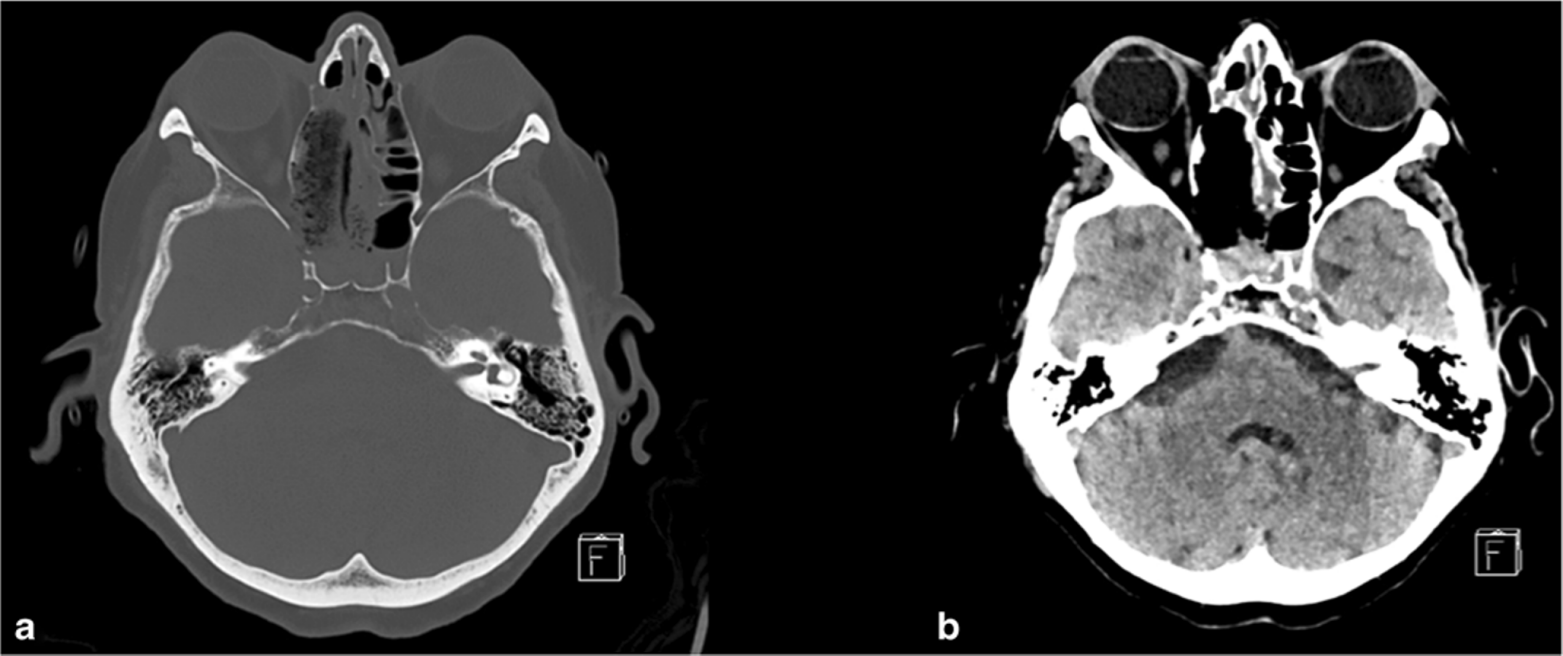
Postoperative CT-imaging after endonasal endoscopic transpterygoidal approach to Meckel’s cave with a bony and b tissue windowing demonstrating the bone access to Meckel’s cave, as well as the defect after extended endonasal transpterygoidal approach with right side turbinectomy, uncinectomy, posterior ethmoidectomy and wide sphenotomy
